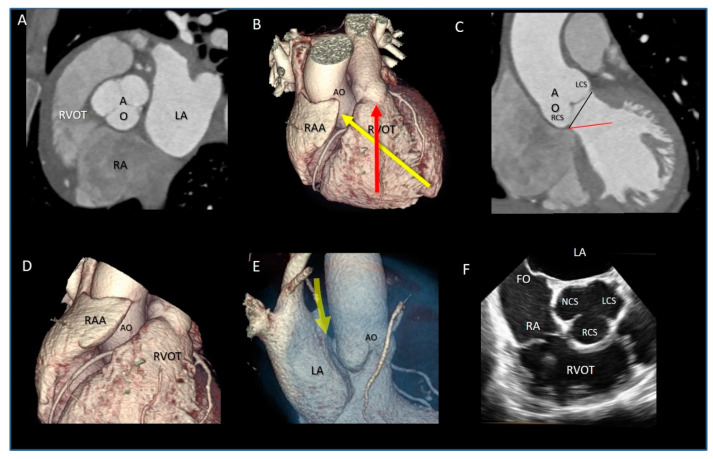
(A) Computed tomographic image in multiplanar modality, showing the aortic root (AO) is the cardiac centerpiece, surrounded by the atria and the right ventricular outflow tract (RVOT). (B) The 3D volume rendering CT image in antero-posterior projection showing the obliquity of the aortic root (yellow arrow), sited posterior and rightward to the RVOT (red arrow). (C) Computed tomographic in multiplanar imaging modality showing the correct attitudinal orientation of the aorta. The black line, that joins the nadir of aortic sinuses, is near 30° tilted in respect to the horizontal red line, so that the left coronary sinus (LCS) and its leaflet are at the highest position among the right coronary (RCS) and non-coronary sinuses. (D) CT image in 3D volume rendering modality in antero-lateral projection showing the AO is surrounded laterally by the right atrial appendage (RAA). (E) CT image in 3D volume rendering modality in lateral projection, showing the space between the left atrium (LA) and the AO (yellow arrow) named “sinus transversum” filled up by epicardial adipose tissue. (F) The 2D transesophageal echocardiography in short-axis aortic view, showing the close proximity between the fossa ovalis (FO) and the non-coronary sinus (NCS) (see text).
